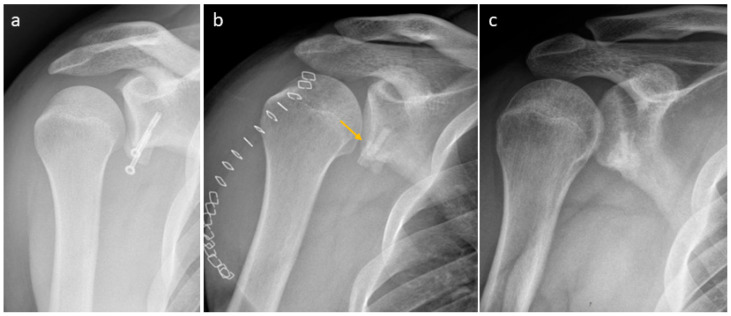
A failed Latarjet in a patient. (a): Pre-surgical X-ray; (b): the metal screws were taken out and the bone bed was prepared for the Shark Screw^®^ (drilling and thread cutting). One Shark Screw^®^ (orange arrow) was used for revision. Union was observed two months after surgery. (c): An X-ray taken four months after surgery shows that the Shark Screw^®^ is nearly remodelled into the host bone.
